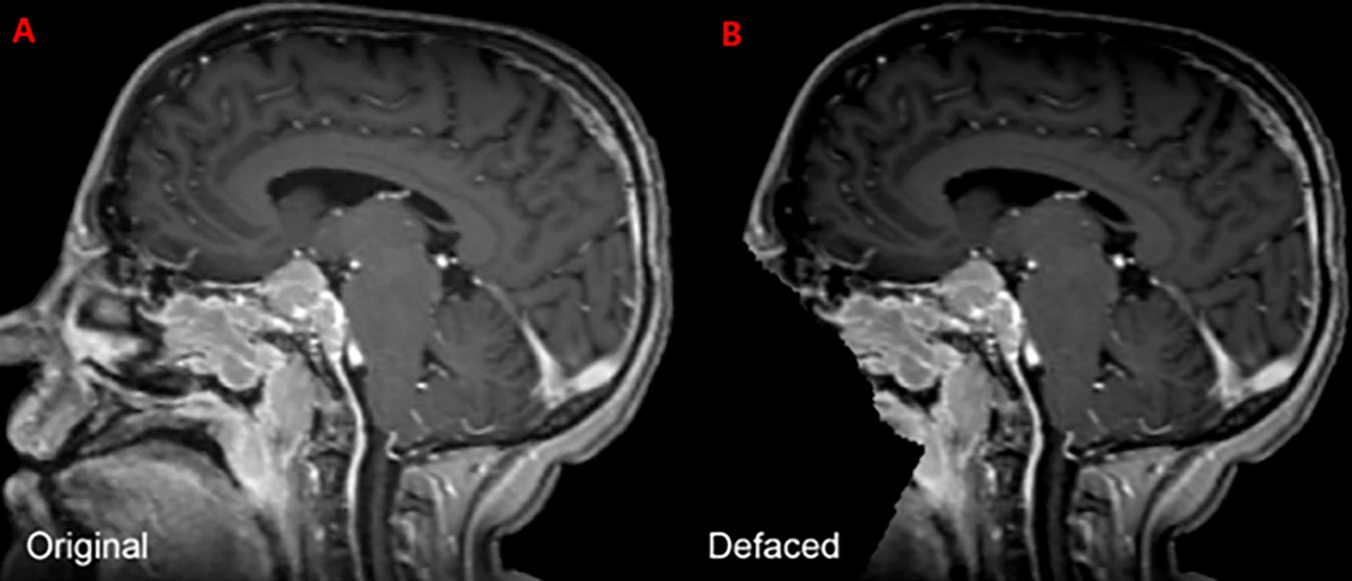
Example of a sagittal image of a brain MRI from the BraTS-MEN-RT training set, both before (A) and after (B) automated defacing.
